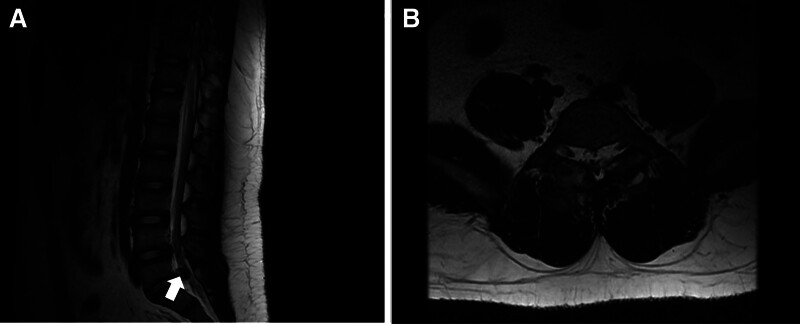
A lumbar spine MRI depicting abnormal signals at L5, slightly reduced signals on T1WI and T2WI, and slightly elevated lipid pressure sequences on T2 (both signals were unclear boundaries, and corresponded to spinal cord compression, indicated by an arrow). MRI = magnetic resonance imaging.
